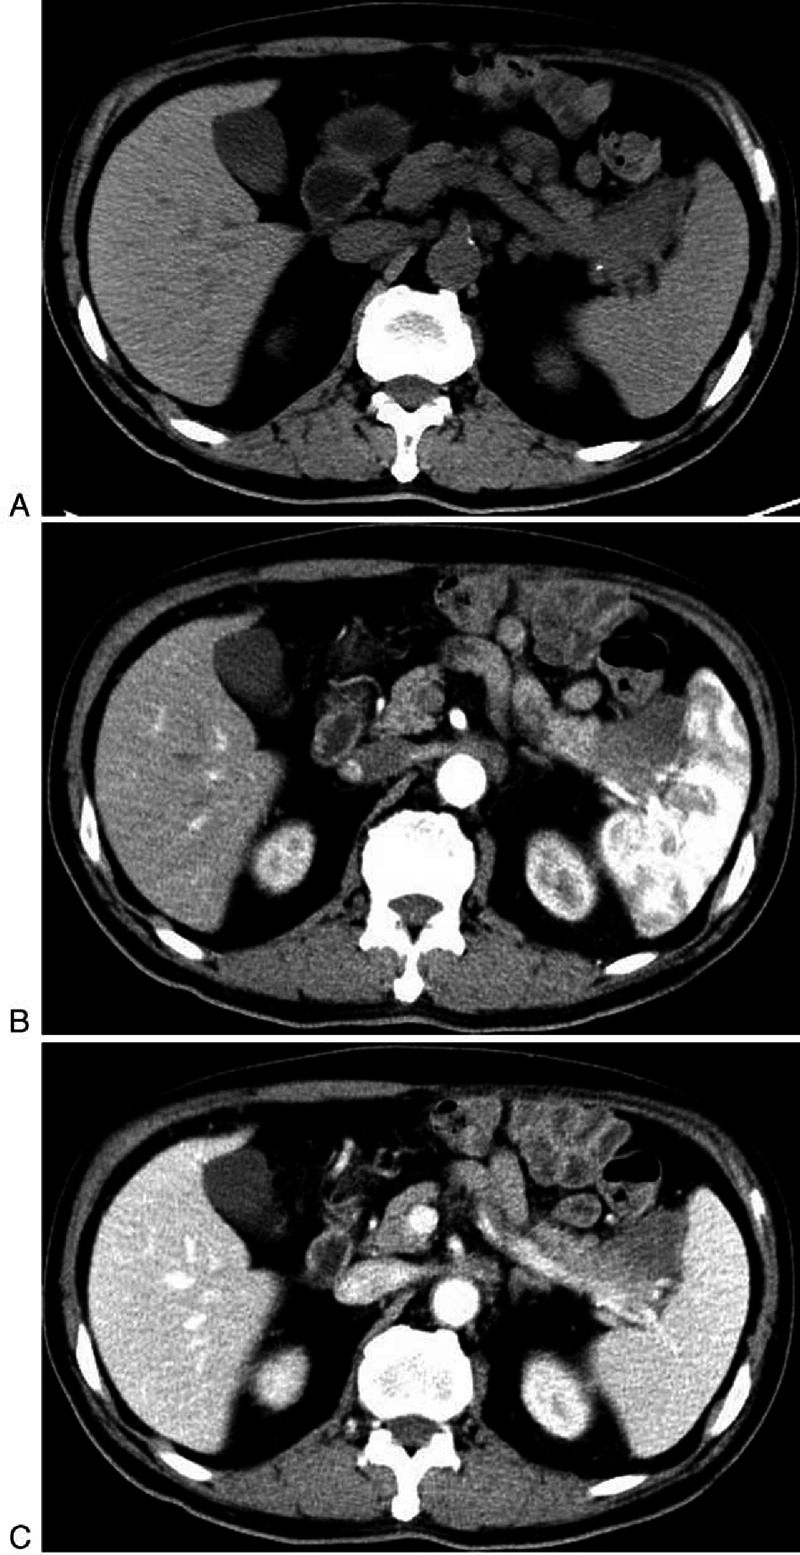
A 63-year-old man with neuroendocrine ethmoid sinus carcinoma (patient 17). A, Unenhanced CT show a lesion in the pancreatic tail exhibite localized pancreatic infiltration instead of focal mass. B-C, Arterial phase and venous phase show mild enhancement of this metastasis.
